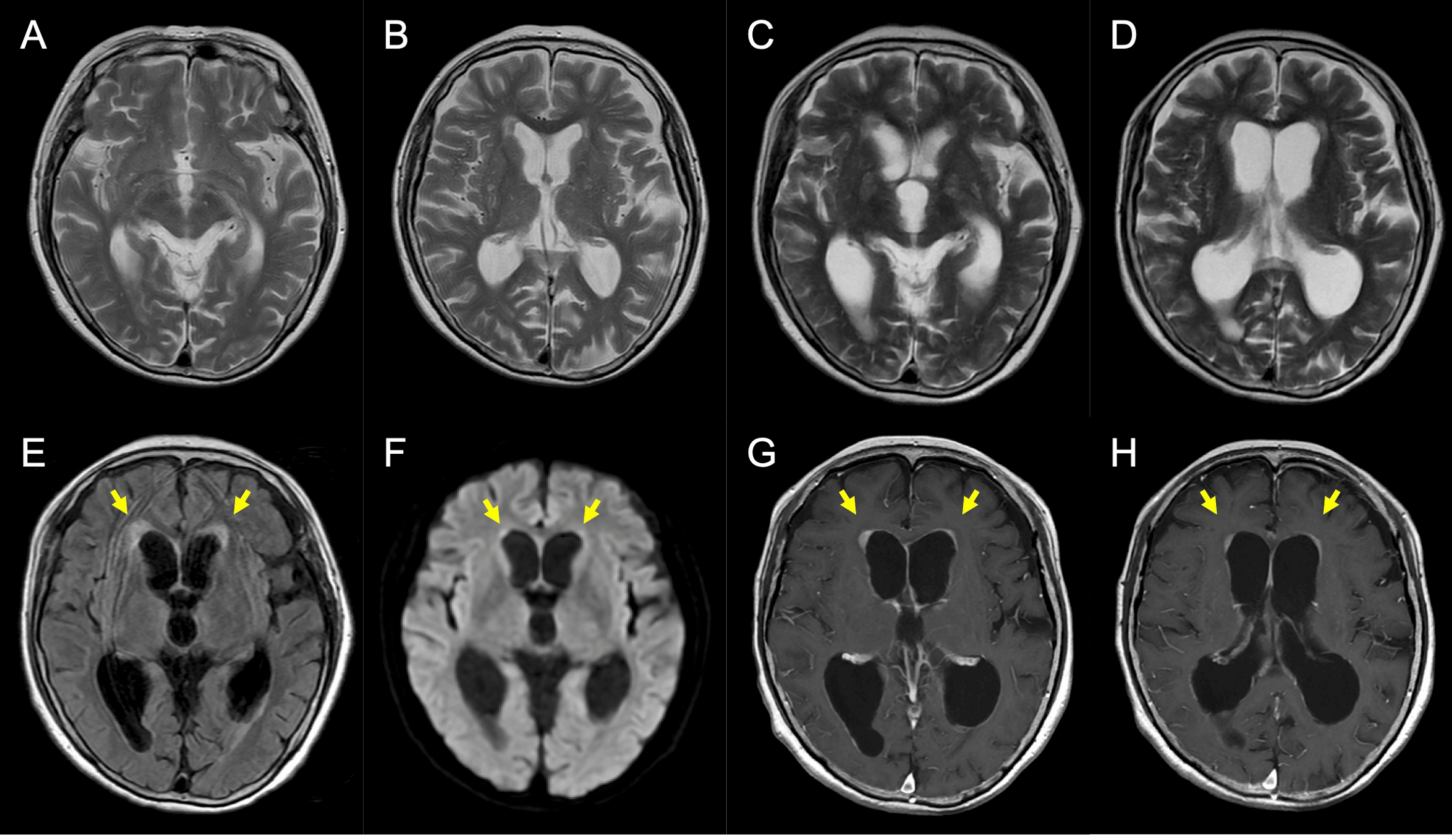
(A,B) T2-weighted images obtained at the local clinic two weeks before the initial visit to our hospital. Sections through the third ventricle (A) and the lateral ventricles (B). (C-H) Magnetic resonance images obtained at our hospital. T2-weighted images (C,D), fluid-attenuated inversion recovery image (E), diffusion-weighted image (F), and contrast-enhanced T1-weighted image (G,H). Each ventricle appears to enlarge in only a two-week period. Yellow arrows indicate abnormal slight hyperintensity areas on fluid-attenuated inversion recovery (E) and diffusion-weighted imaging (F), or faint contrast-enhancement on the T1-weighted image (G, H). Sections through the third ventricle (C,E,F,G) and the lateral ventricles (D,H)
